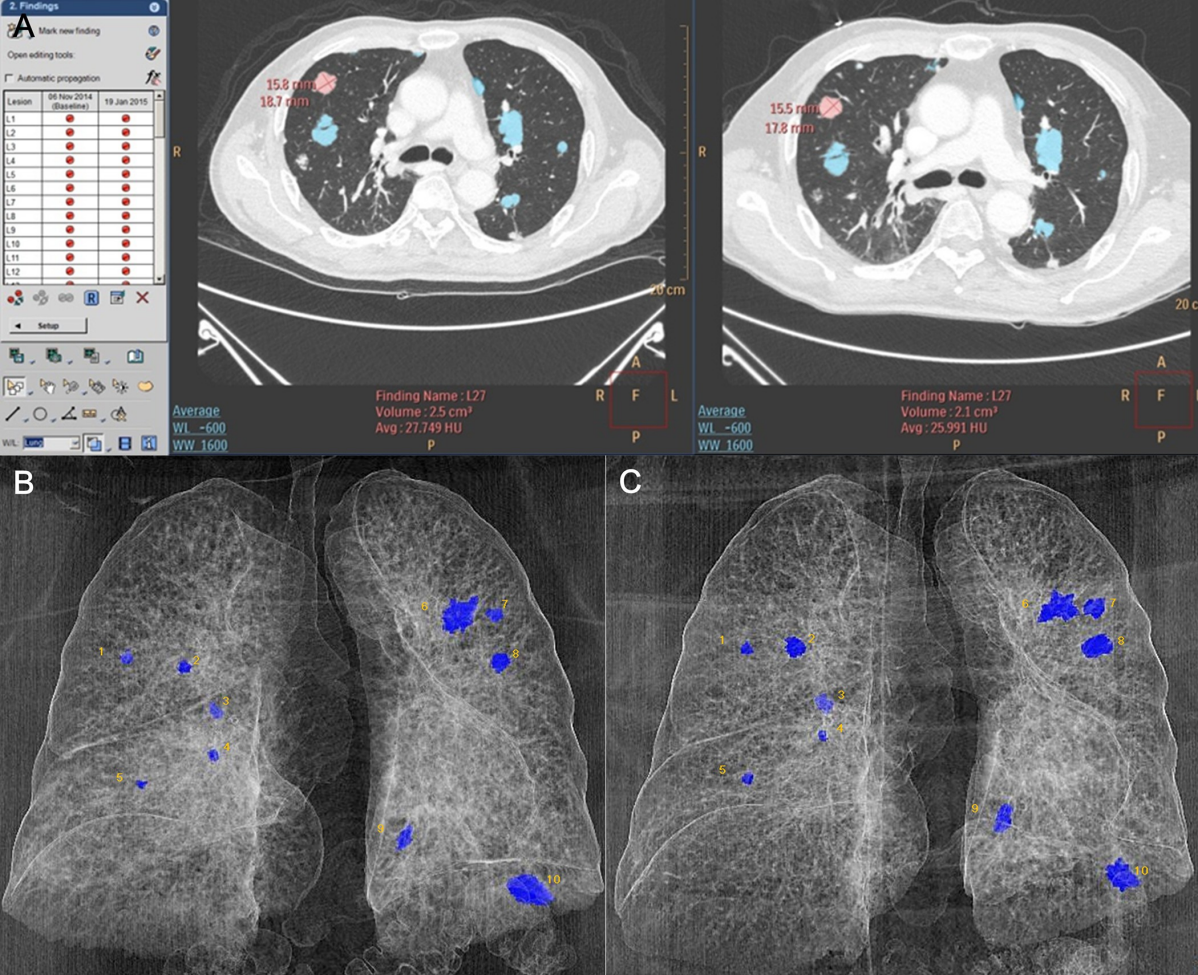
Lesion segmentation and tumor tracking by semi-automated 3D CT volumetry of multiple pulmonary metastatic nodules. (a) Metastatic pulmonary nodules are automatically segmented and manually edited on axial CT images of two time points using 3D visualization software. After segmentation, the 3D maximum diameter and volume of each nodule are automatically calculated. (b, c) Segmented pulmonary metastatic nodules are seen in 3D reconstructed images of baseline (b) and follow-up (c) chest CT scans.
